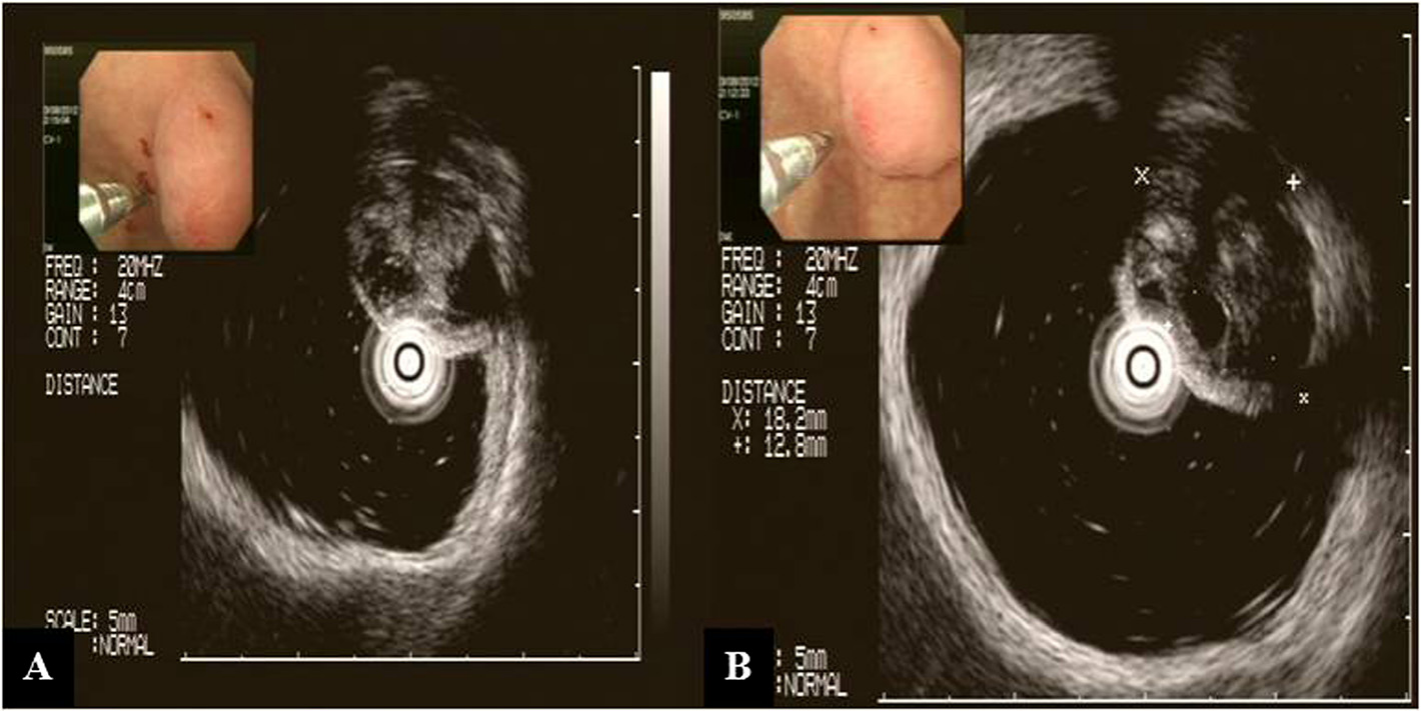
Endoscopic ultrasonographic findings (A) and (B). The lesion seems to originate from the third layer with heterogeneous echogenicity and an approximately 18mm focally cystic center.
